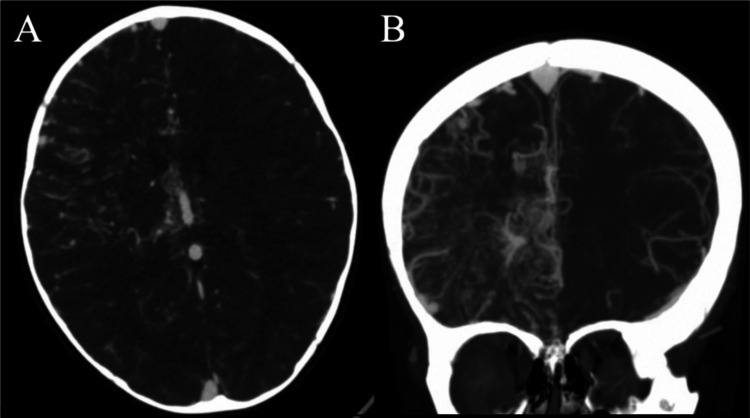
Initial computed tomography angiogram. A Axial and B coronal sequences showing increased vascularity in the right hemisphere concerning for a vascular malformation
